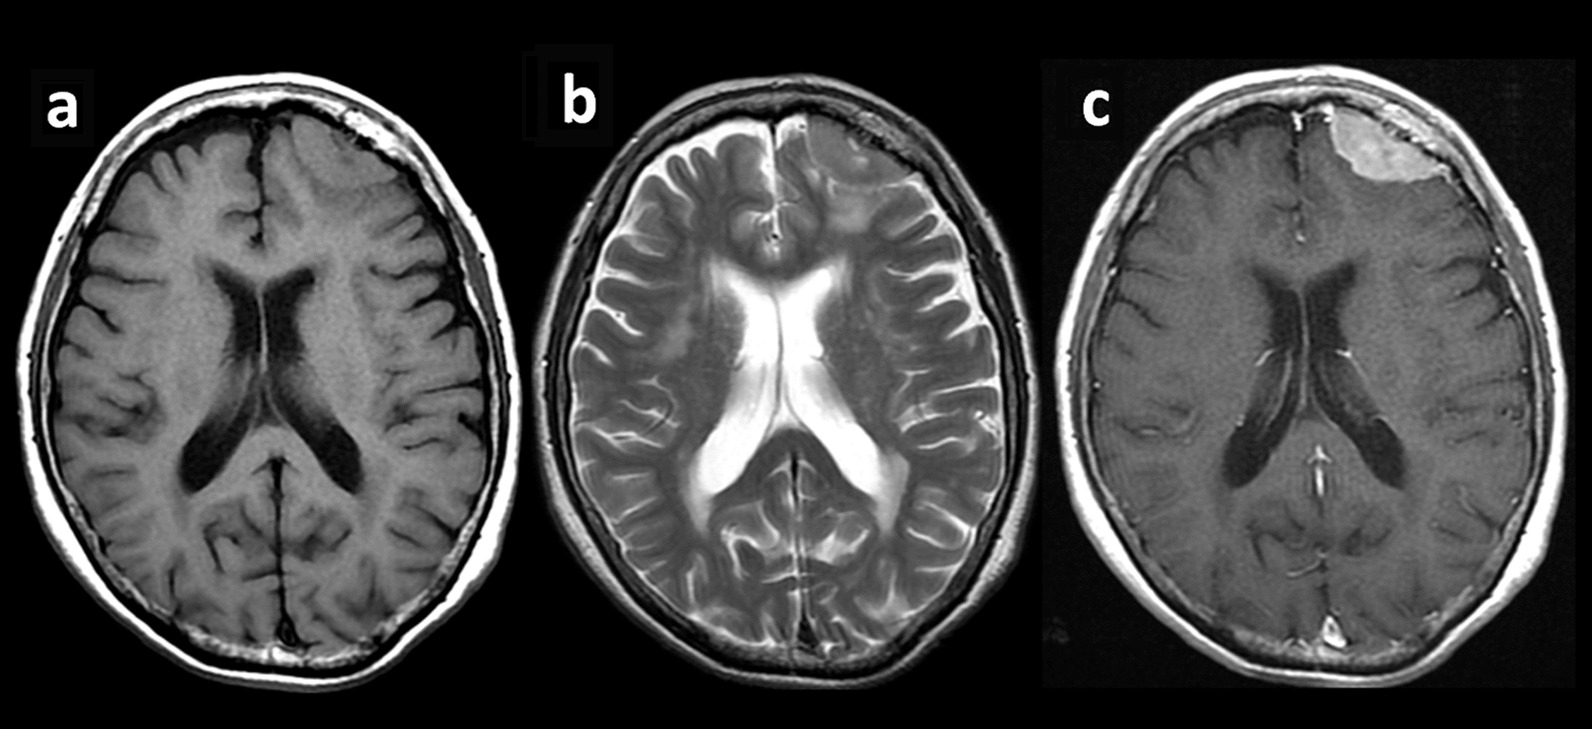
Preoperative magnetic resonance imaging shows an extra-axial mass in the left frontal lesion. Post-contrast T1 weighted images show an enhancing dural-based mass. a The axial T1-weighted image. b The axial T2-weighted image. c The axial contrast-enhanced image
